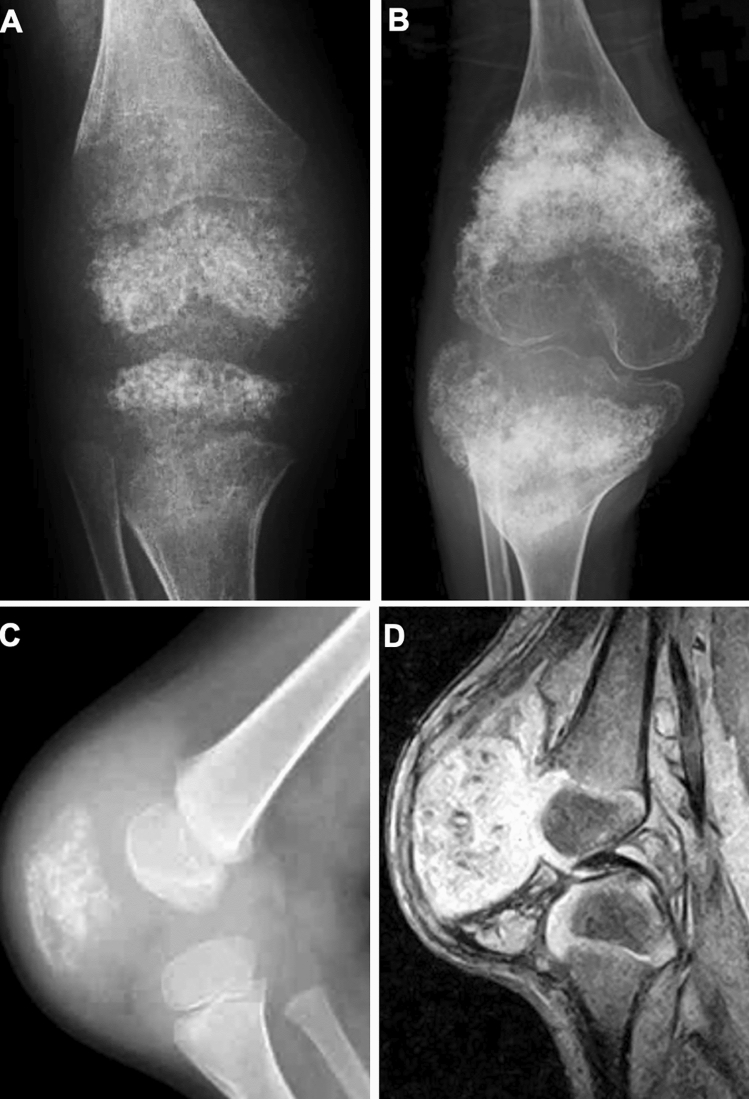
Two cases with CINCA. A–B. A child with a long history of systemic illness. The frontal radiographs of the knees show age-dependent evolution of epi-metaphyseal cartilaginous overgrowth with abnormal ossifications at age 2 years (A) and 14 years (B). C-D. A different child. The lateral radiograph of the knee shows characteristic patellar overgrowth with abnormal ossifications (C). Sagittal gradient echo image shows a markedly enlarged patellar cartilage with the same signal intensity as an articular cartilage, associated with low signal spots reflecting internal calcification (D)
